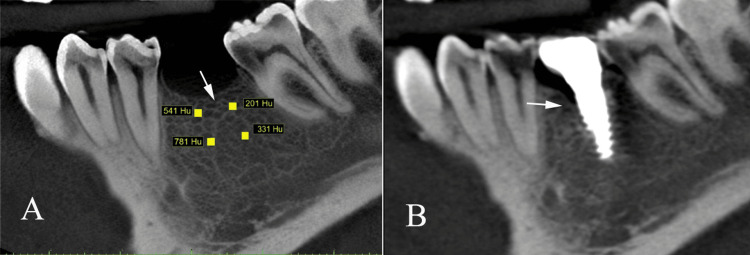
CBCT images The images show (A) preoperative measurements of bone density (HU) at the planned implant site (white arrow) and (B) the postoperative site with the implant placed (white arrow) CBCT: cone-beam computed tomography
